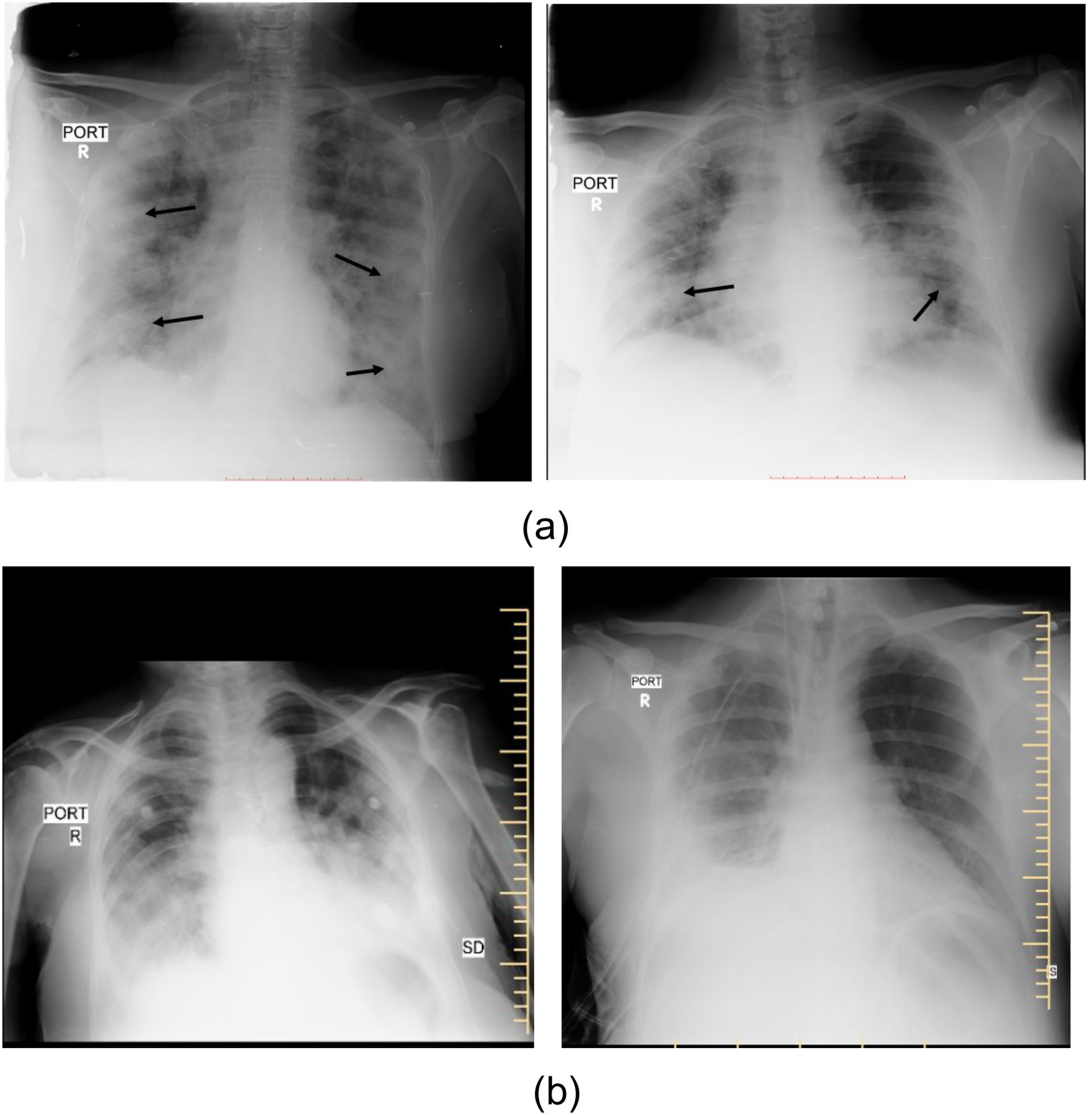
Chest X-rays of (a) RT-PCR proven COVID-19 pneumonia in cases showing the typical bilateral peripheral consolidations and ground-glass opacities. (b) Non-COVID-19 pneumonia in cases showing the lobar distribution of consolidations with pleural effusion.
